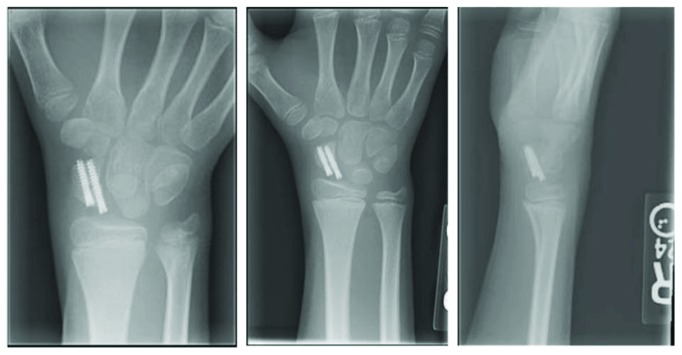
AP and lateral radiographs taken two weeks postsurgical fixation using two Arthrex micro screws.
